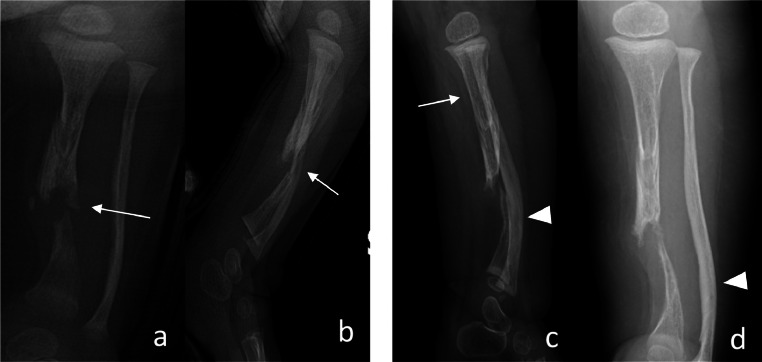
X-rays performed after 2 months (A and B) and after 8 months (C and D) show absence of osseous consolidation of the fracture (white arrows). (C and D) Along with fracture, tibial erosion is visible in its proximal tract (white arrow); fibula appears intact, but with significant antero-lateral bowing (arrowhead).
